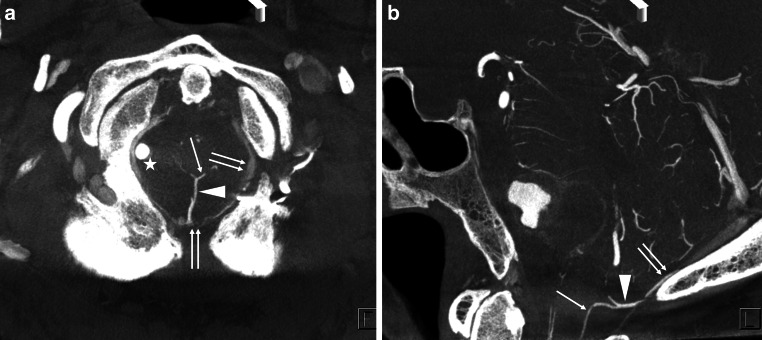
A representative case of the cerebellomedullary cistern (CMC) bridging vein (BV). Axial (a) and sagittal (b) view of the slab MIP images from contrast-enhanced cone-beam CT show that the transverse medullary vein (*arrow* in (a)) and posterior spinal vein (*arrow* in (b)) drain into the marginal sinus (*double*
